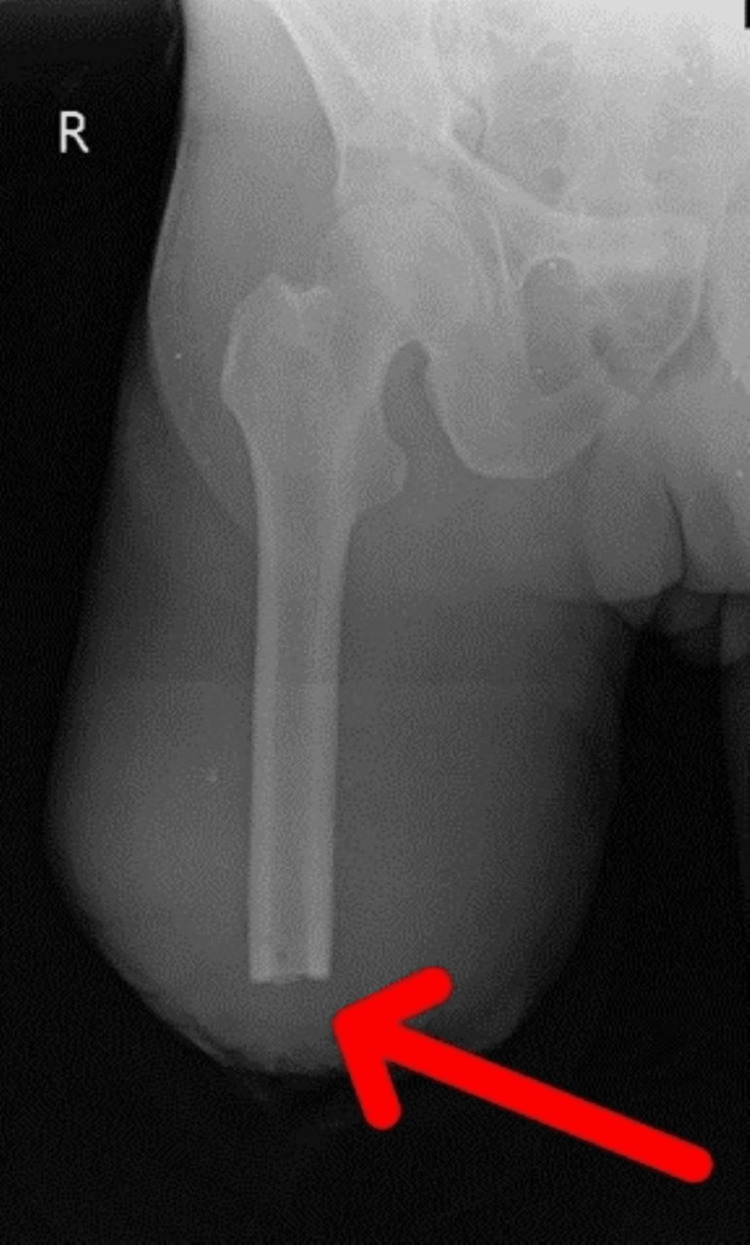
Post-operative X-ray showing above-knee amputation (red arrow).
